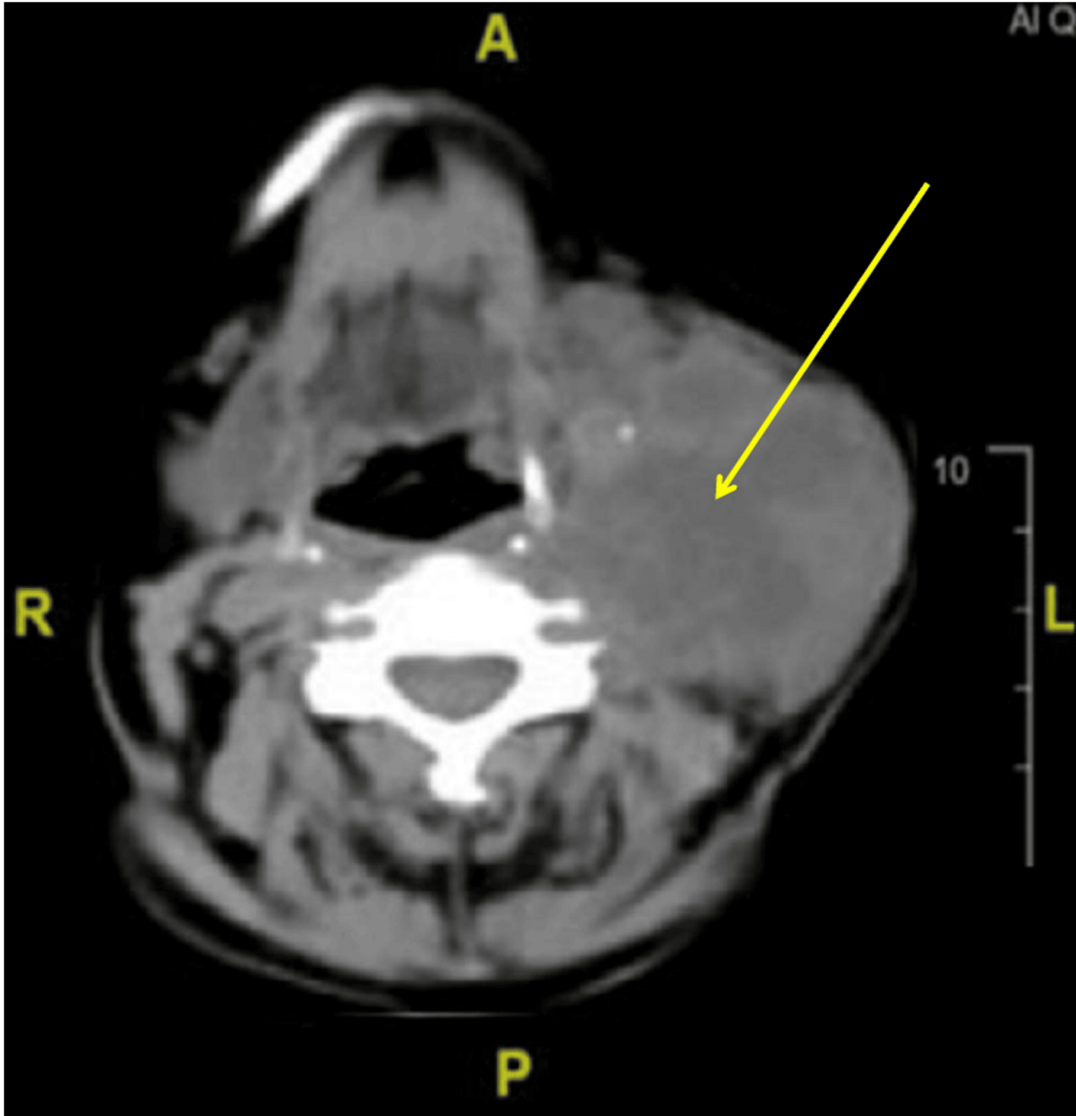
Contrast-enhanced CT of the neck showing a heterogeneous solid mass in the left lateral neck (yellow arrow) CT: computed tomography
